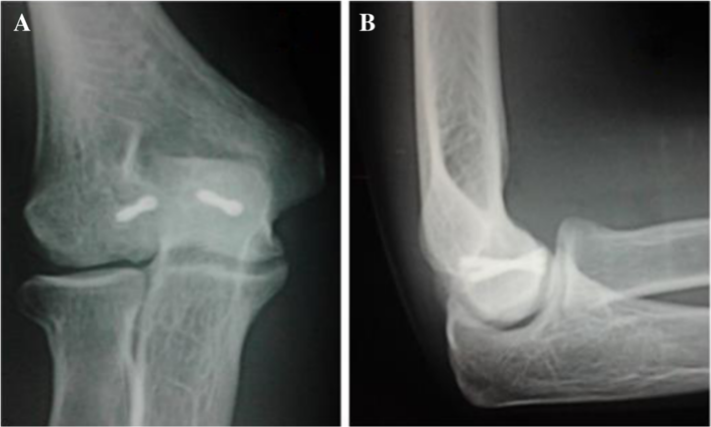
Radiograph after two years of follow-up. (A) Anteroposterior view and (B) lateral view.
